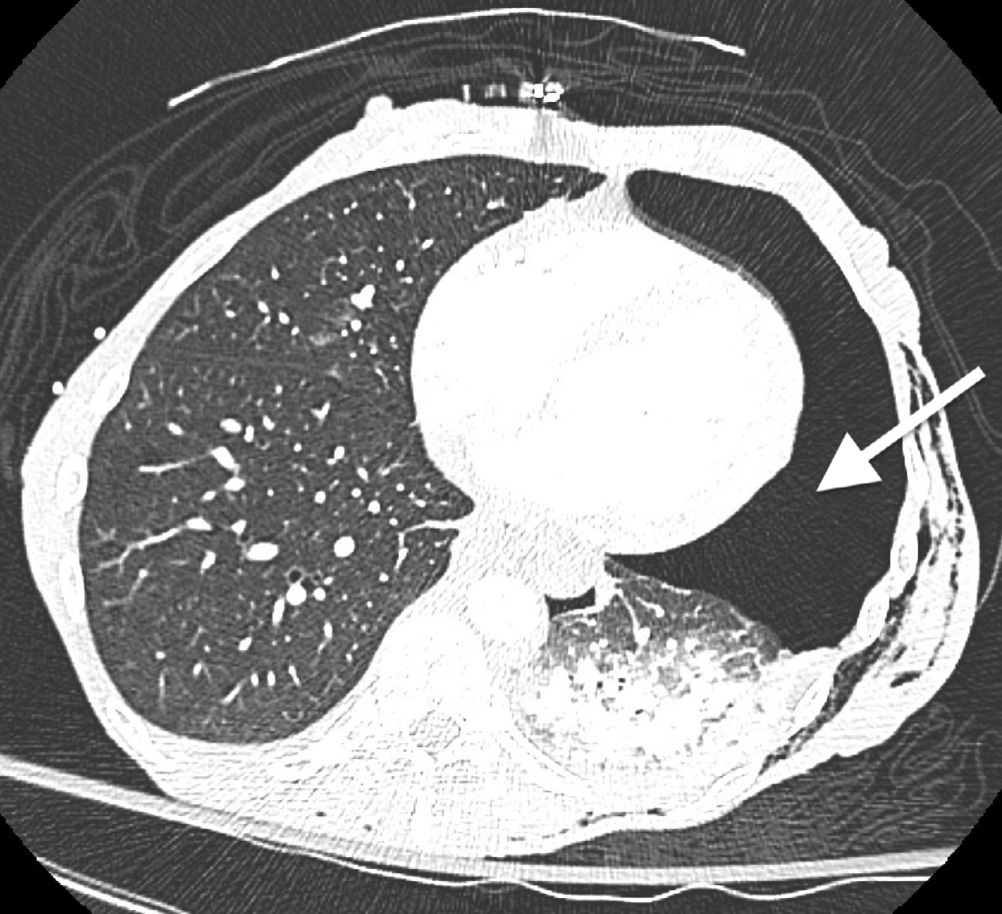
Axial CT image of the chest demonstrating large left sided pneumothorax (white arrow).
